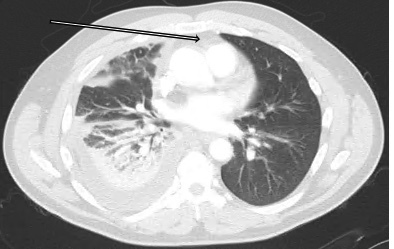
Computed tomography angiogram of the chest. Arrow pointing at an encapsulated mediastinal fatty lesion with soft tissue stranding. Other notable findings include sympathetic right pleural effusion with right lung atelectasis.
